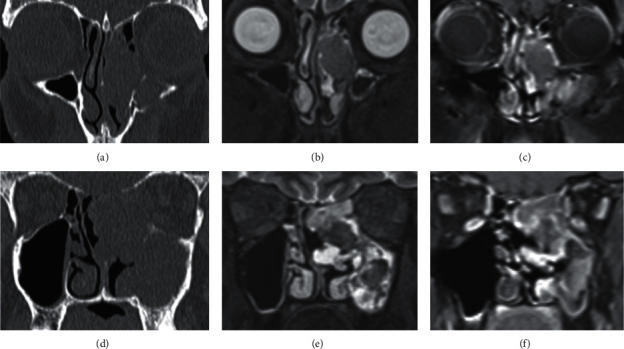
Diffuse large B-cell lymphoma in a 60-year-old male with a 4-month history of nasal blockage not responding to antibiotics. (a) Coronal unenhanced CT shows left sinonasal opacification with thinned medial maxillary sinus wall and destroyed middle turbinate. (b) Coronal STIR demonstrates a left nasal cavity lesion obstructing the maxillary sinus, which is hypointense to mucosa with heterogenous enhancement on (c) contrast-enhanced T1W. The left cribriform plate appears thinned and focally dehiscent on unenhanced CT (d); however, (e) STIR and (f) contrast-enhanced T1W show the skull base and medial orbital wall are intact.
